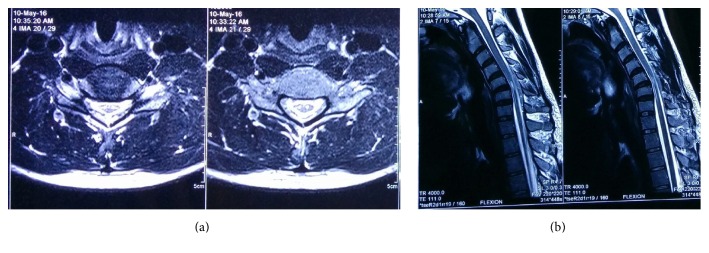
(a) MRI showing focal symmetrical atrophy of spinal cord with intramedullary T2 hyperintensity. (b) MRI showing forward displacement of the posterior subdural sac on flexion of neck, with prominent epidural space.
